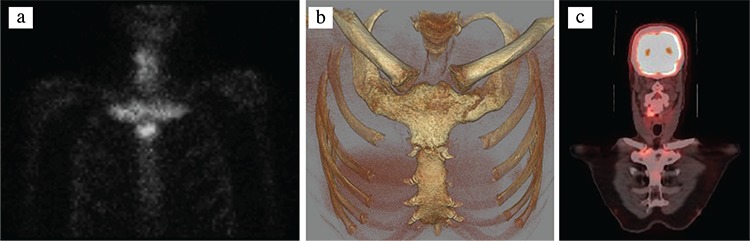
a-c. Anterior view of ^99m^Tc-methylene diphosphonate bone scintigraphy (a) showing increased osteoblastic activity in the manubriosternal, bilateral sternocostoclavicular (bull’s head sign), and lower cervical vertebral regions. Computed tomographic three-dimensional reconstruction of the bones of the anterior chest wall showing hyperostosis and fusion of the first costosternal and manubriosternal joints (b) and oblique coronal ^18^F-fluorodeoxyglucose positron emission tomography–computed tomography fusion image (c) showing increased activity in sternoclavicular joints (arthritis) and hyperostotic lesions (osteitis).
